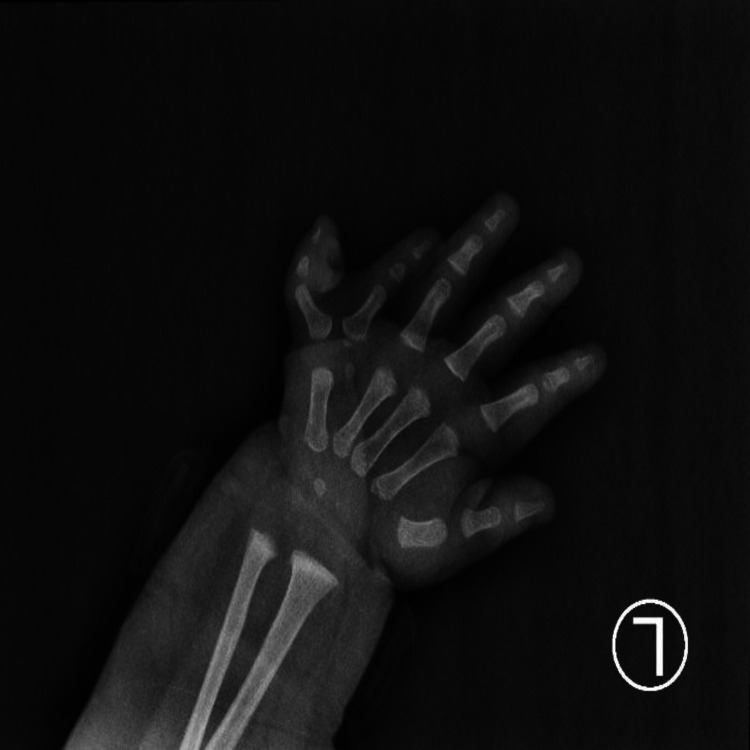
X-rays of the patient’s left hand showing postaxial polydactyly (Stelling and Turek type 2)
